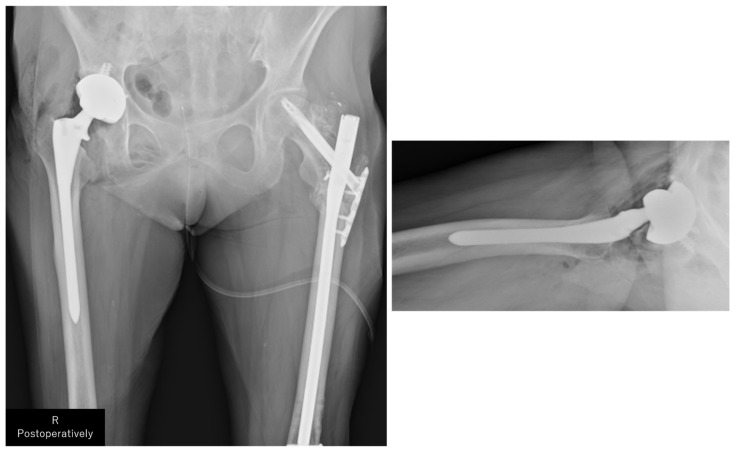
Plain radiograph of the hip joint immediately postoperatively. The stem (Arcos^®^ One-piece Femoral Revision System, Zimmer, Warsaw, IN, USA, φ9.5 mm × 175 mm, high offset) was inserted to a depth of 11 mm below the apex of the greater trochanter, as planned preoperatively. The cup (Continuum^®^, Zimmer, Warsaw, IN, USA, Φ28/46 mm) was fixed and positioned using four screws. The surgery was completed in 1 h 20 min, with a blood loss of 50 mL.
